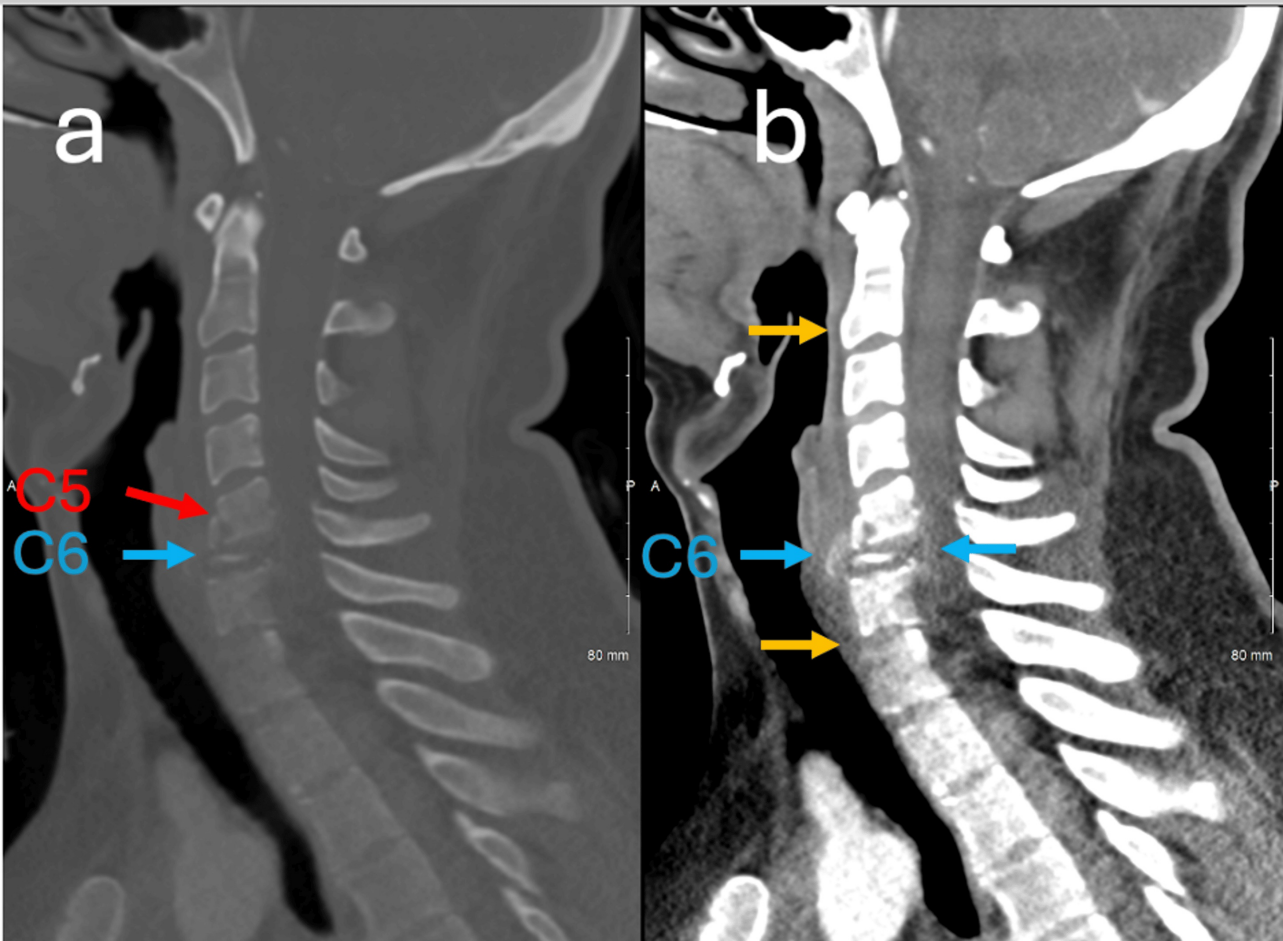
Sagittal bone and soft tissue window CT demonstrating C6 vertebral body destruction from metastatic paraganglioma with pathologic fracture and prevertebral hematoma. (a) Sagittal CT of the cervical spine with bone window demonstrates significant destructive changes of the C6 vertebral body secondary to proven metastatic paraganglioma (blue arrow) as well as flexion teardrop fracture with retrolisthesis of the C5 vertebral body with respect to the C6 upper endplate (red arrow). (b) Sagittal CT of the cervical spine with soft tissue window demonstrates C6 vertebral body collapse with circumferential expansion (blue arrows) resulting in canal narrowing. A small amount of prevertebral hematoma is also present (light orange arrows).
